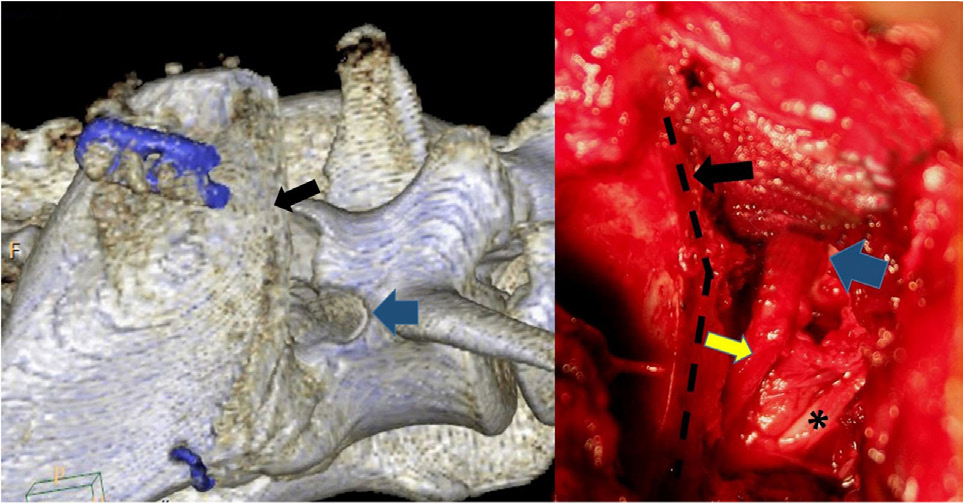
The osteotomy (black arrow and black dotted line) has been repeated digitally on the postoperative CT scan to mimic the patient’s intraoperative procedure. The blue arrows mark the cranial rim of the enlarged lumbosacral foramen, while the L6 nerve exhibits a normal structure (*). The L7 nerve (yellow arrow) has been freed of adhesions. The visible part of the nerve is increased in thickness.
